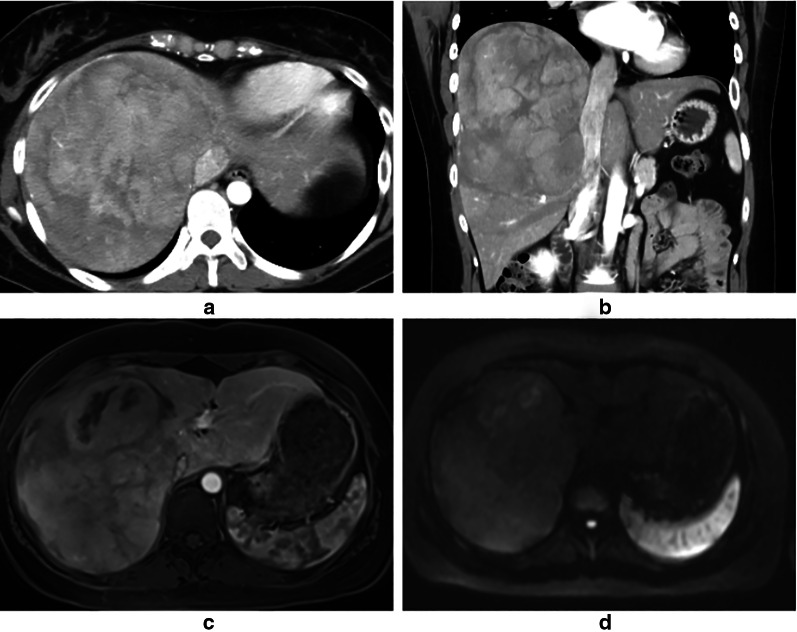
Enhanced computed tomography (CT) and gadolinium ethoxybenzyl diethylenetriamine pentaacetic acid-enhanced MRI findings CT showed a 150-mm-diameter tumor in the right hepatic lobe: a axial view, b coronal view. MRI showed c. No high intensity is detected compared to the background liver in the diffusion-weighted images (d)
